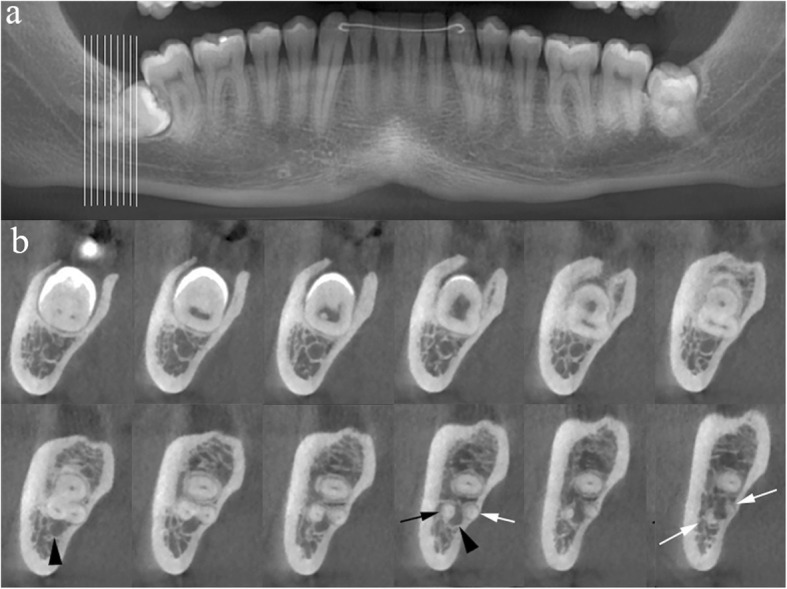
Anatomical variant of nerve course. CBCT of the mandible, panorex reconstruction (a) indicating the range of the cross-sectional images in b. The roots (arrows) of the included right third molar encompass and remodel the mandibular canal (arrowheads), increasing the risk of nerve damage during tooth extraction
